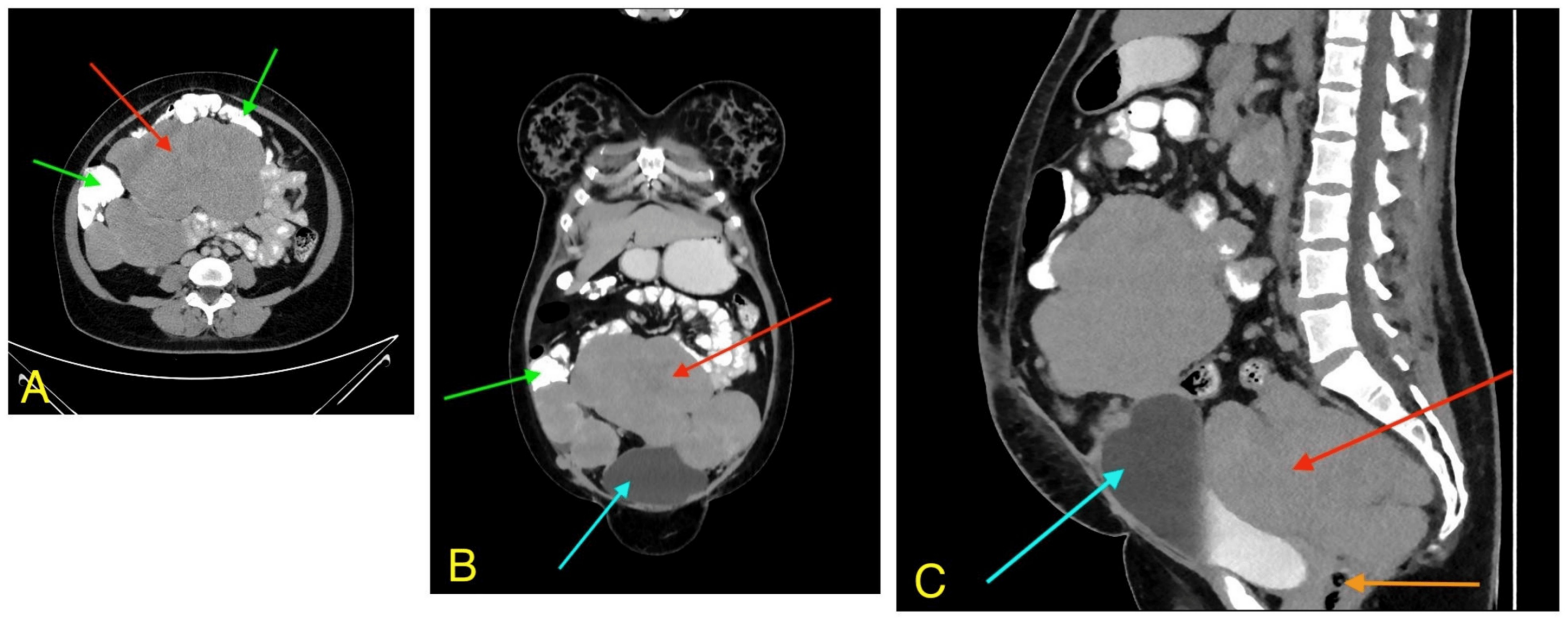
CT scan images of the abdomen – the axial (A), coronal (B), and sagittal (C) sections CT, Computerized tomography. (A) - The axial section shows the small bowel (green arrows) splayed by the large tumor (red arrow) occupying the whole of the abdomen. (B) - The coronal section shows a large central tumor (red arrow) pushing the small bowel (green arrow) up and out and abutting the urinary bladder (blue arrow). (C) - The sagittal section shows the pelvic component of the tumor (red arrow) pushing the urinary bladder (blue arrow) in the front, and compressing the rectosigmoid junction (orange arrow).
